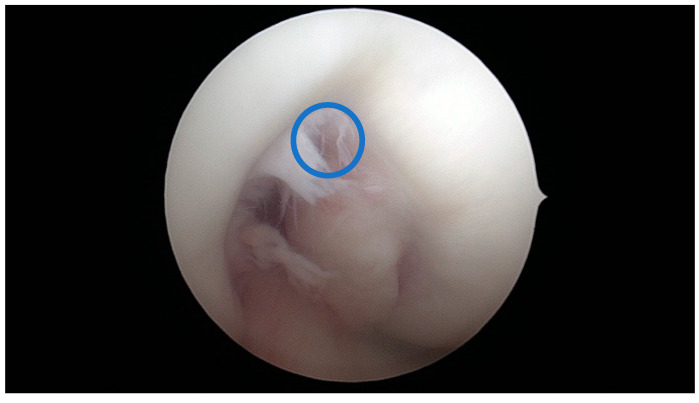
A-type notch; blue circle = aICN area measured on the MRI.
